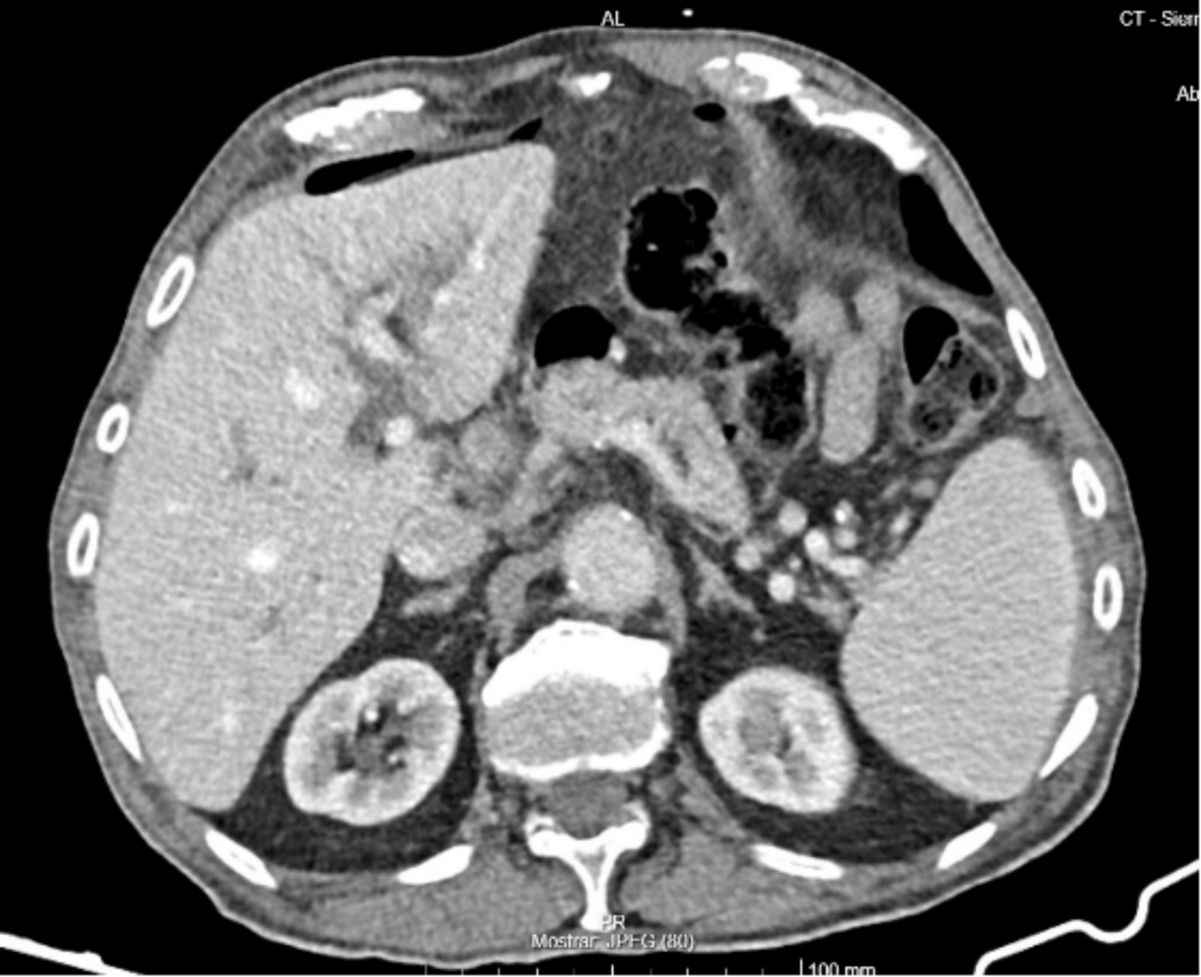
CT scan evidence of pneumoperitoneum
